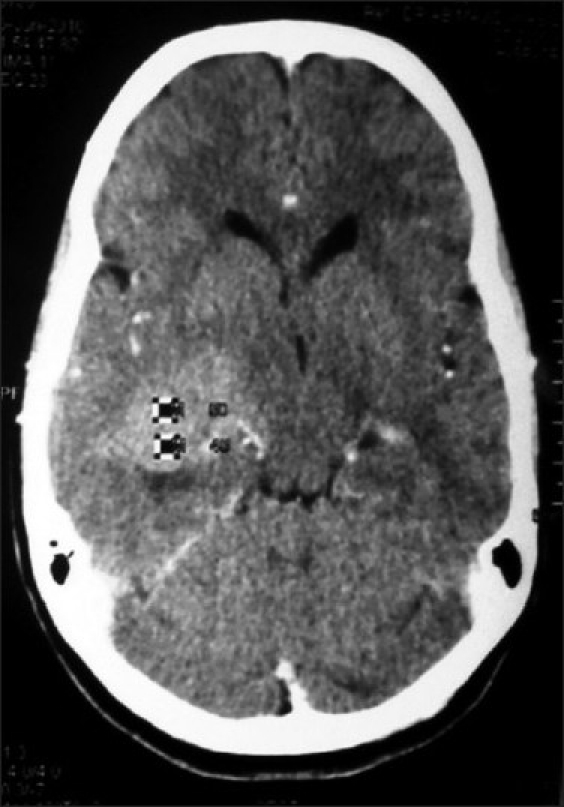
Right diencephalic oligodendroglioma on contrast CT-scan brain in a 13 year old sister, whose 10 year brother (Figure 6) has fourth ventricular ependymoma
